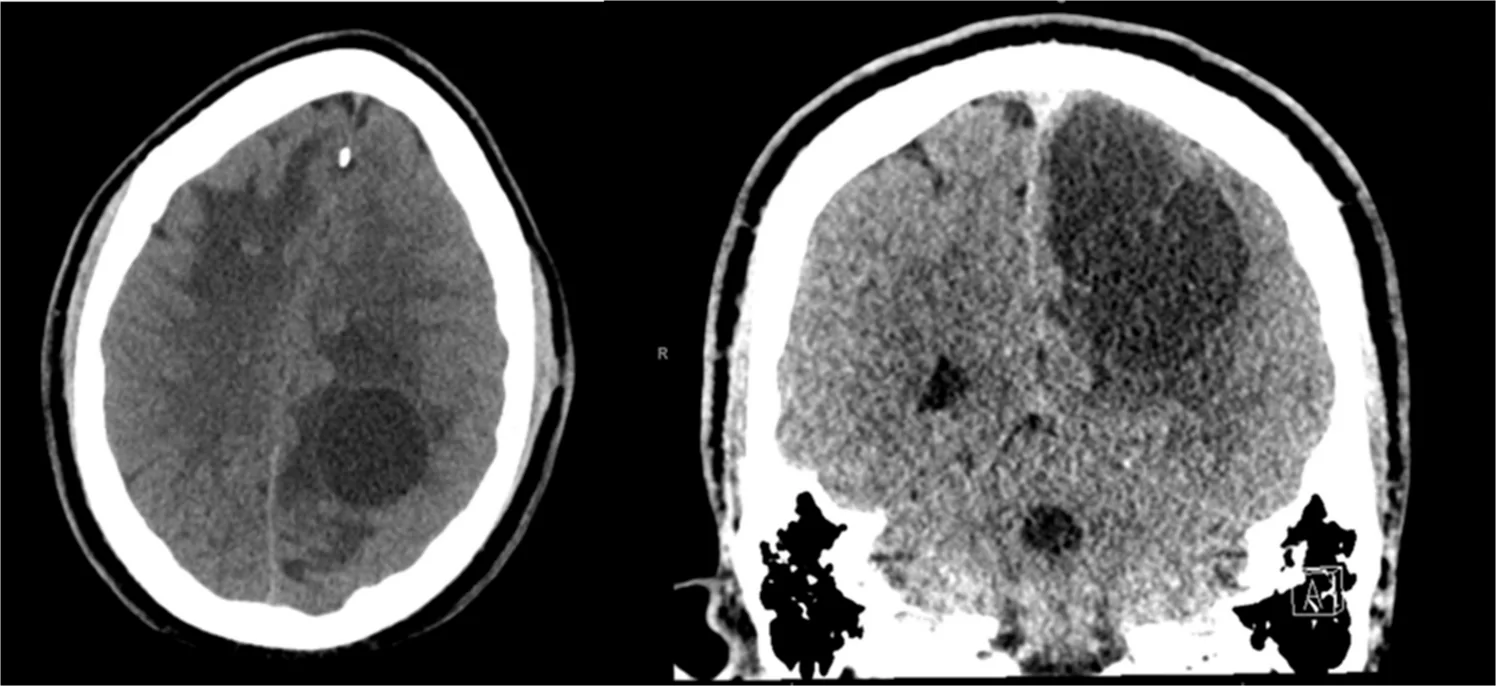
Patient’s initial CT head without contrast with a heterogenous parieto-occipital lesion with a 3.8-cm cystic component and a 3-cm solid component and a hyperdense 1.1-cm solid right frontal centrum semiovale lesion with significant surrounding vasogenic edema best demonstrated in the left image
